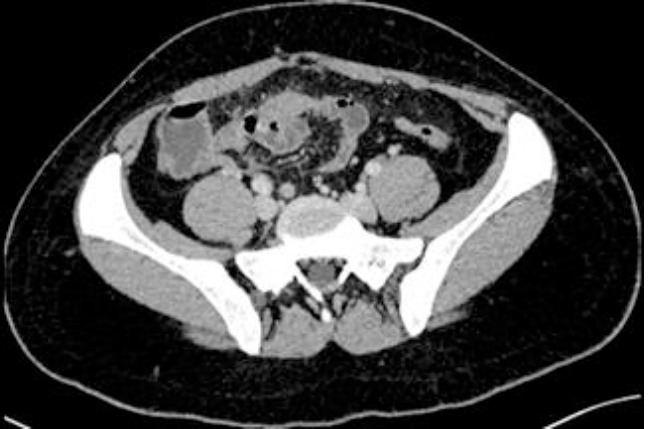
normal appearance of the appendix in a computed tomography scan
